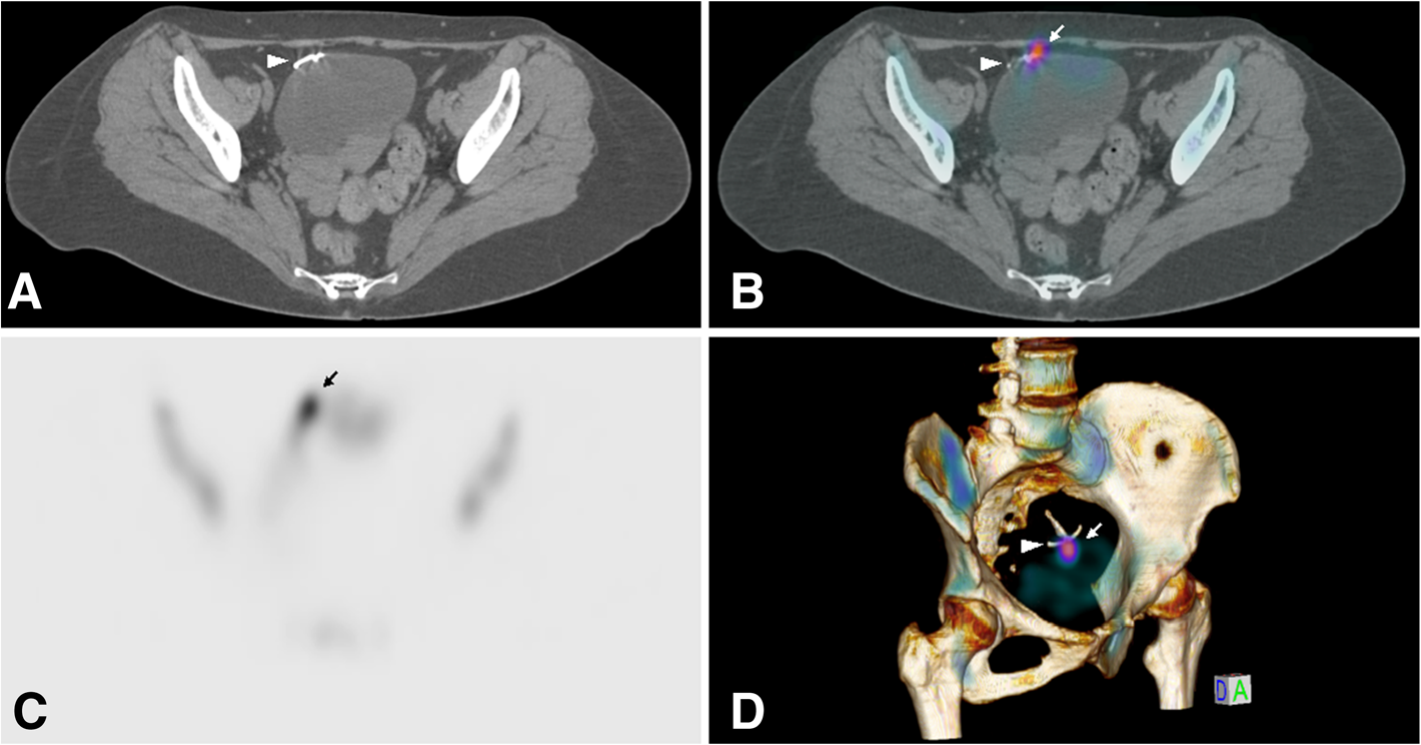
Pelvic single photon emission computed tomography with computed tomography reveals a focal ^99m^Tc-hydroxymethylene diphosphonate hyperfixation (plain arrow) centered at a mislocated intrauterine device (arrow head) in the anterior vesical wall. (A) Axial computed tomography slice. (B) Axial single photon emission computed tomography. (C) Axial single photon emission computed tomography with computed tomography. (D) Volume-rendering single photon emission computed tomography with computed tomography.
